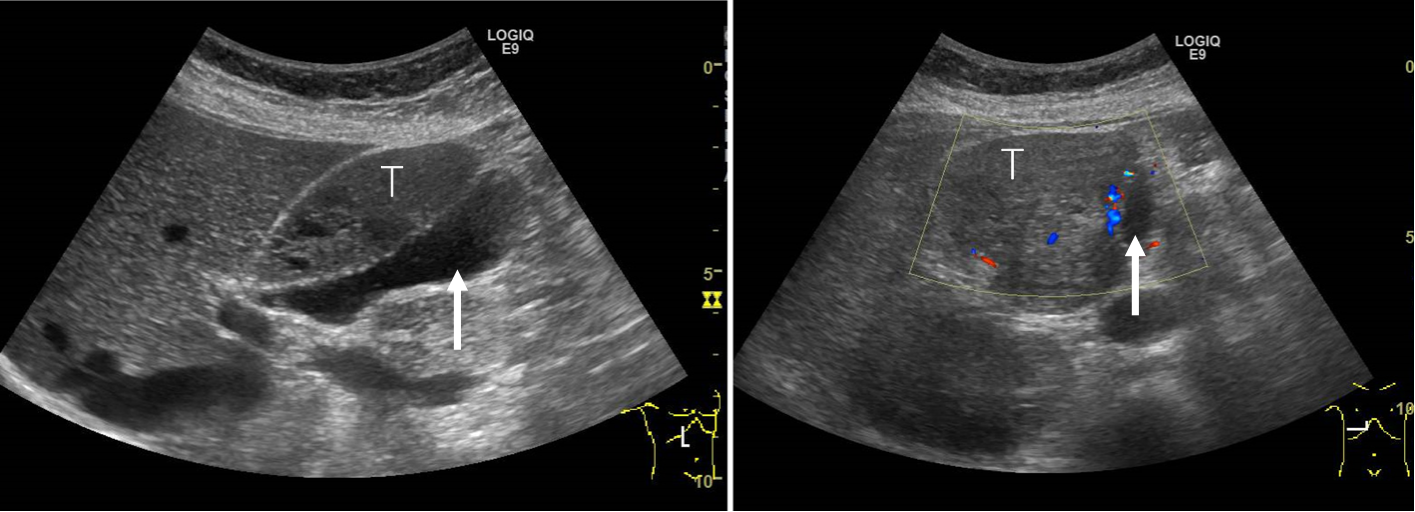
Abdominal ultrasonography showed a tumor (T) with a clear border between the gallbladder (white arrow) and the liver
